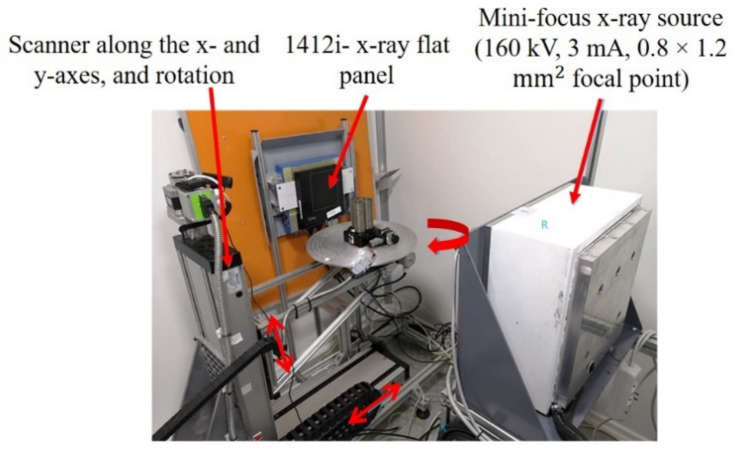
Mini-focus X-ray equipment for radiographic analyses.
